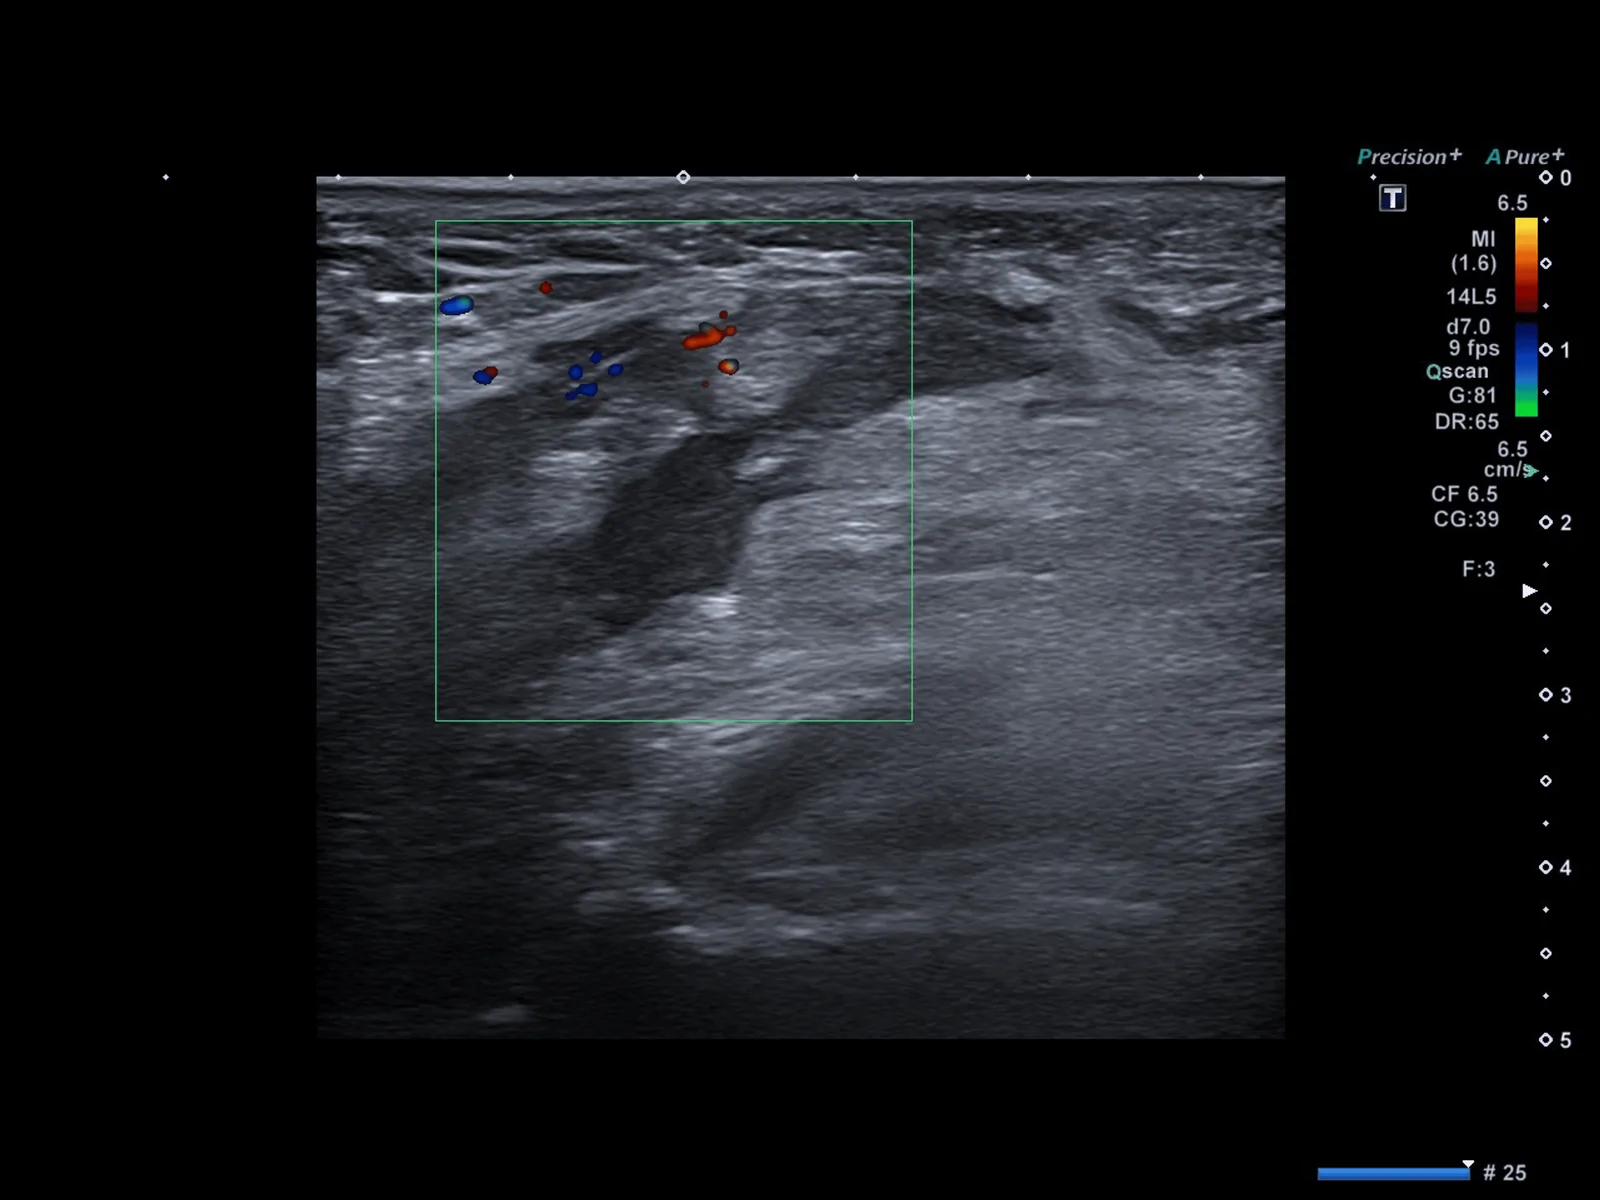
Ultrasound examination of the left inguinal region Color Doppler ultrasonography demonstrating a non-reducible left inguinoscrotal fat-containing lesion with preserved internal vascular flow and an estimated neck diameter of approximately 7 mm, initially interpreted as an incarcerated recurrent inguinal hernia. Surgical exploration subsequently demonstrated that the lesion corresponded to an abscess adjacent to the vas deferens rather than a recurrent inguinal hernia.
